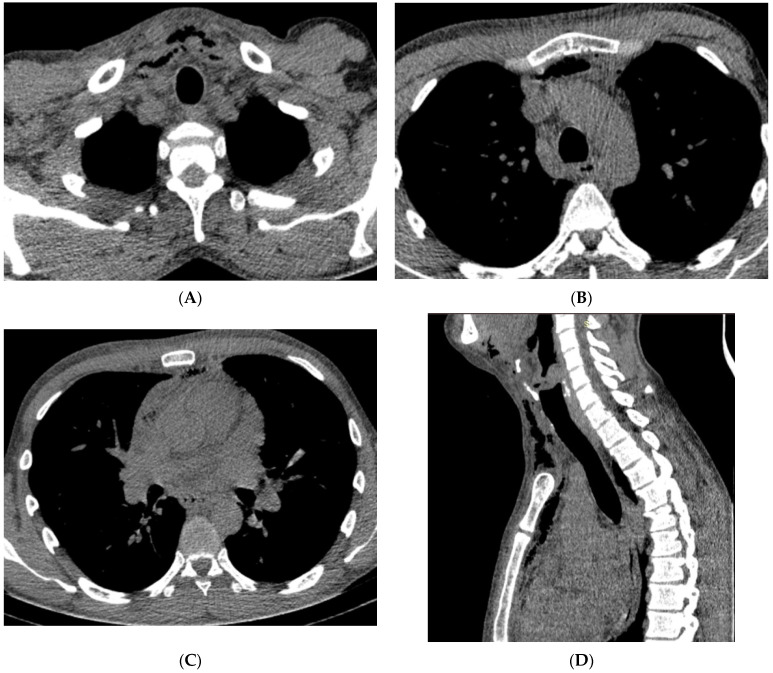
(Patient 3) Axial contrast-enhanced CT image; (A) air collection in the costoclavicular space, (B,C) air collection in the subclavian space, (D) air collection in the anterior mediastinum.
